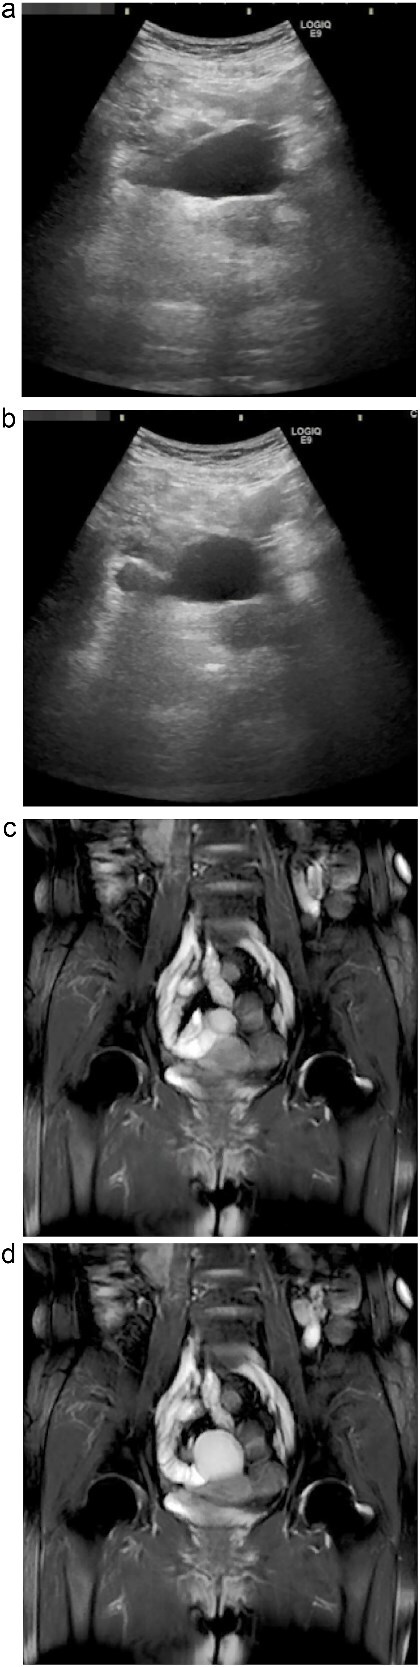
Dynamic ultrasonography and MRI of the abdominal cystic lesion. (a, b) Abdominal ultrasonography revealed a 6-cm cystic lesion with a layered wall structure. Image (b) was taken 4 s after (a), showing deformation of the cyst wall, indicating peristaltic motion. (c, d) Coronal T2-weighted MRI demonstrated a high-intensity cystic lesion adjacent to the ileum. Image (d) was obtained 1.5 s after (c), also showing peristaltic movement of the cyst wall. These dynamic imaging features suggested a duplication cyst with smooth muscle activity.
